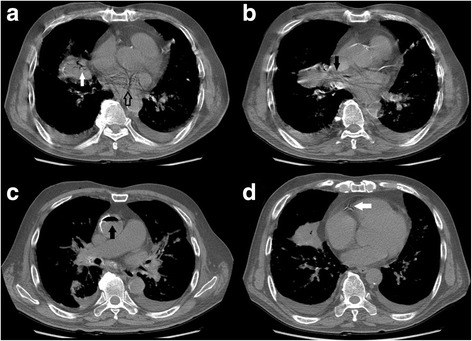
Series of computed tomography scans demonstrating the movement of the air emboli. Immediate post-procedure computed tomography scans demonstrated free air with flow-related motion artifact in a the right middle lobe mass (white arrow), and the left atrium (open arrow), and b the right pulmonary vein (black arrow). After 50 s, a follow-up computed tomography scan demonstrated air collections in c the ascending aorta (black arrow) and d right coronary artery (white arrow)
